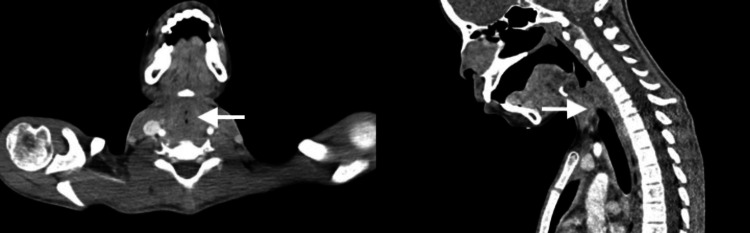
Axial and sagittal computed tomography images showing subglottic stenosis
